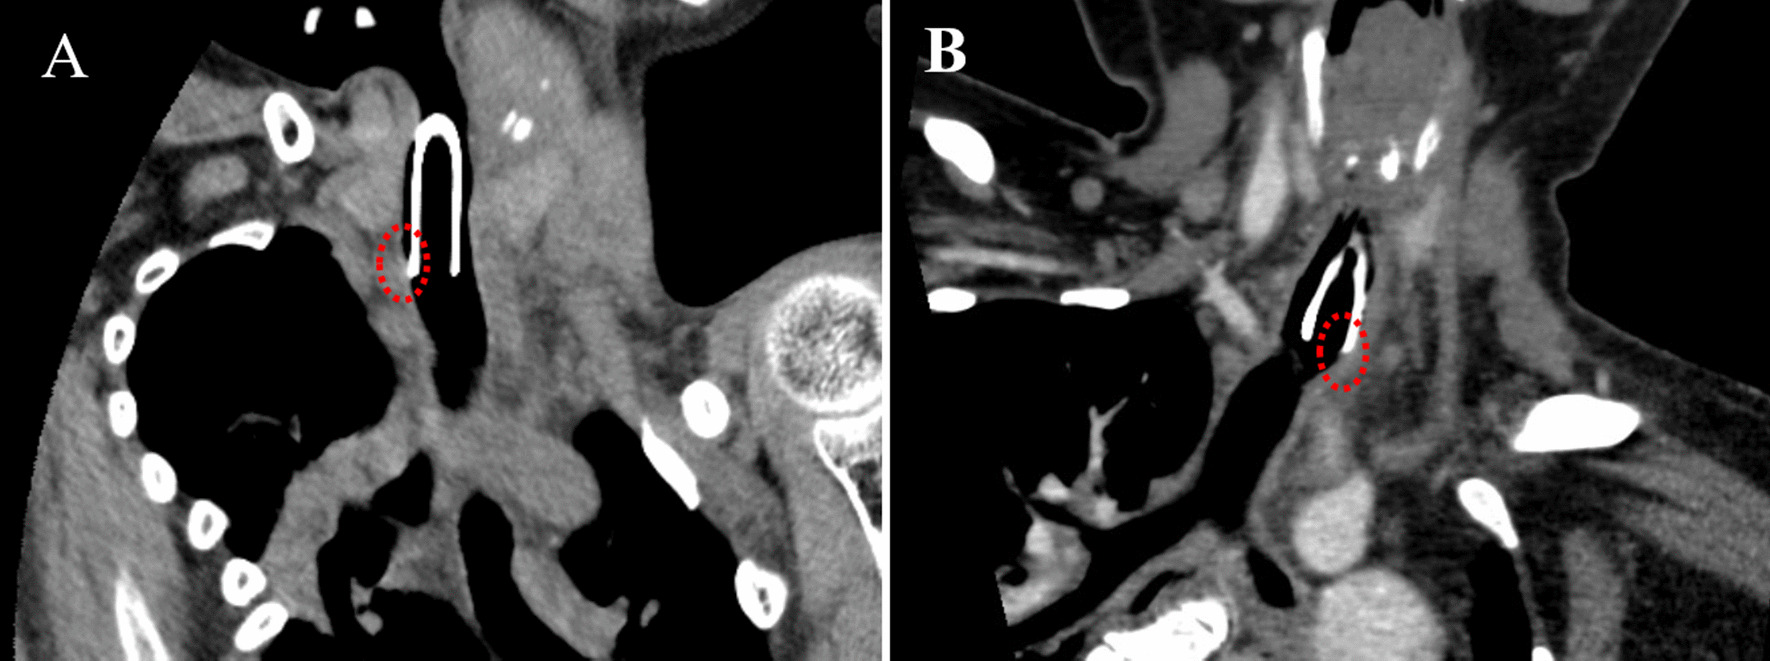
Coronal view of CT showing that the tip of the tracheostomy tube made contact with the trachea due to deformity of the trachea in cases of severe scoliosis (A: case 23, B: case 26). In these cases, the tracheal tube needed shortening
